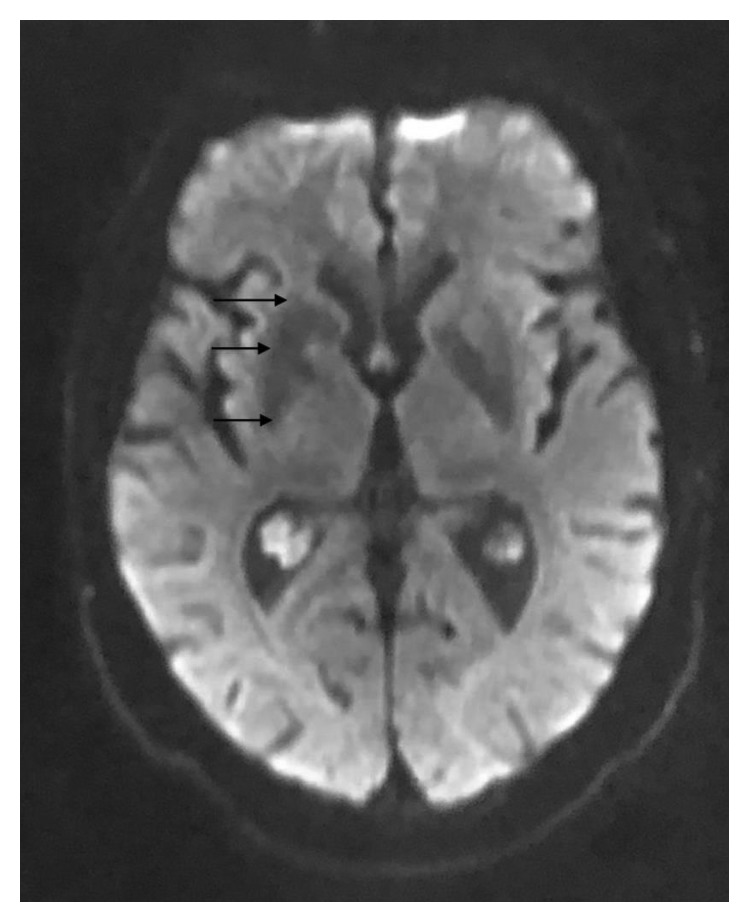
MRI brain with diffusion-weighted axial image. Arrows highlight the corresponding right basal ganglia with no hyperintense signal abnormality to suggest ischemia.
